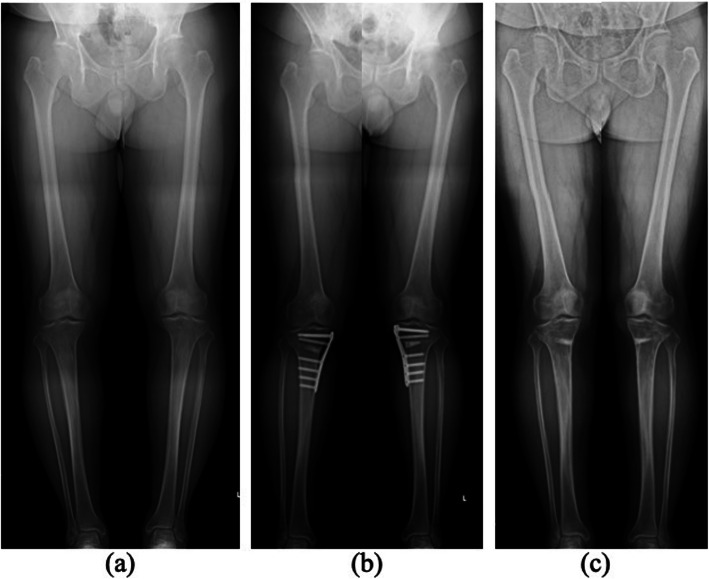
(a) Radiographical examination using a standing full weight-bearing lower extremity teleroentgenogram revealed varus knee angulation with a hip–knee–ankle (HKA) angle of − 2° on the right and − 4° on the left. (b) HKA angles were + 4° valgus on the right and + 5° valgus on the left 1 year after bilateral OWHTO. (c) Alignments of the lower extremities were preserved without correction loss 3 years after bilateral OWHTO and 2 years after the removal of fixations
